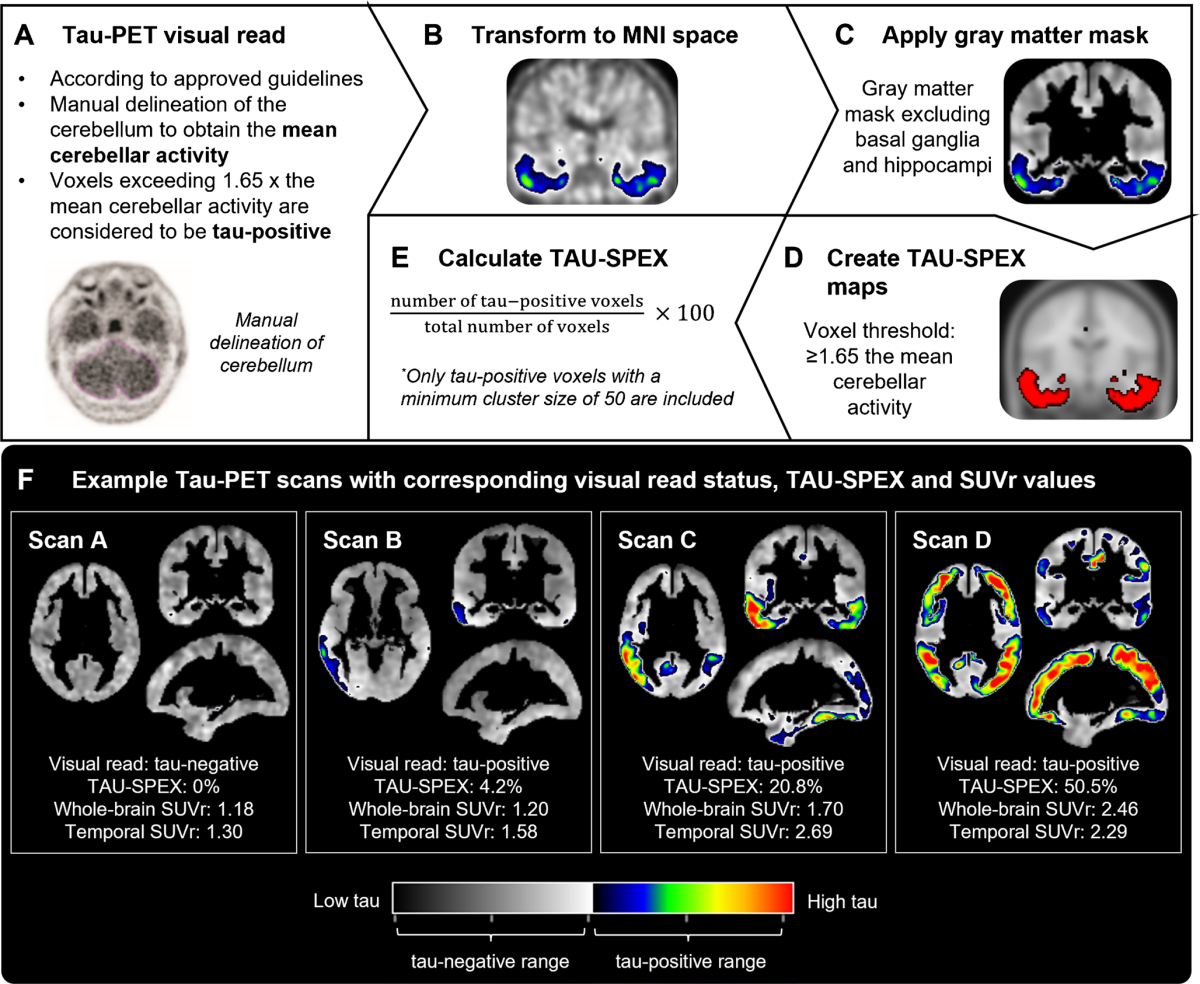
TAU-SPEX methodology. In A, the FDA-approved guidelines for [^18^F]flortaucipir visual read are summarized. Tau-PET images were visually read in native space independently of TAU-SPEX or SUVr. In B-E, our methodology for quantifying TAU-SPEX is shown. TAU-SPEX was developed to closely align with the existing Tau-PET visual read framework, specifically, by using a similar cerebellar scaling and threshold approach as used for visual read. In F, four example scans with corresponding visual read status, TAU-SPEX and SUVr values are shown. These example scans highlight the large variance present among visually tau-positive scans (Scan B, C and D) that is well-captured using TAU-SPEX. Moreover, the example scans in A and B highlight that whole-brain Tau-PET uptake is better captured using TAU-SPEX than SUVr
